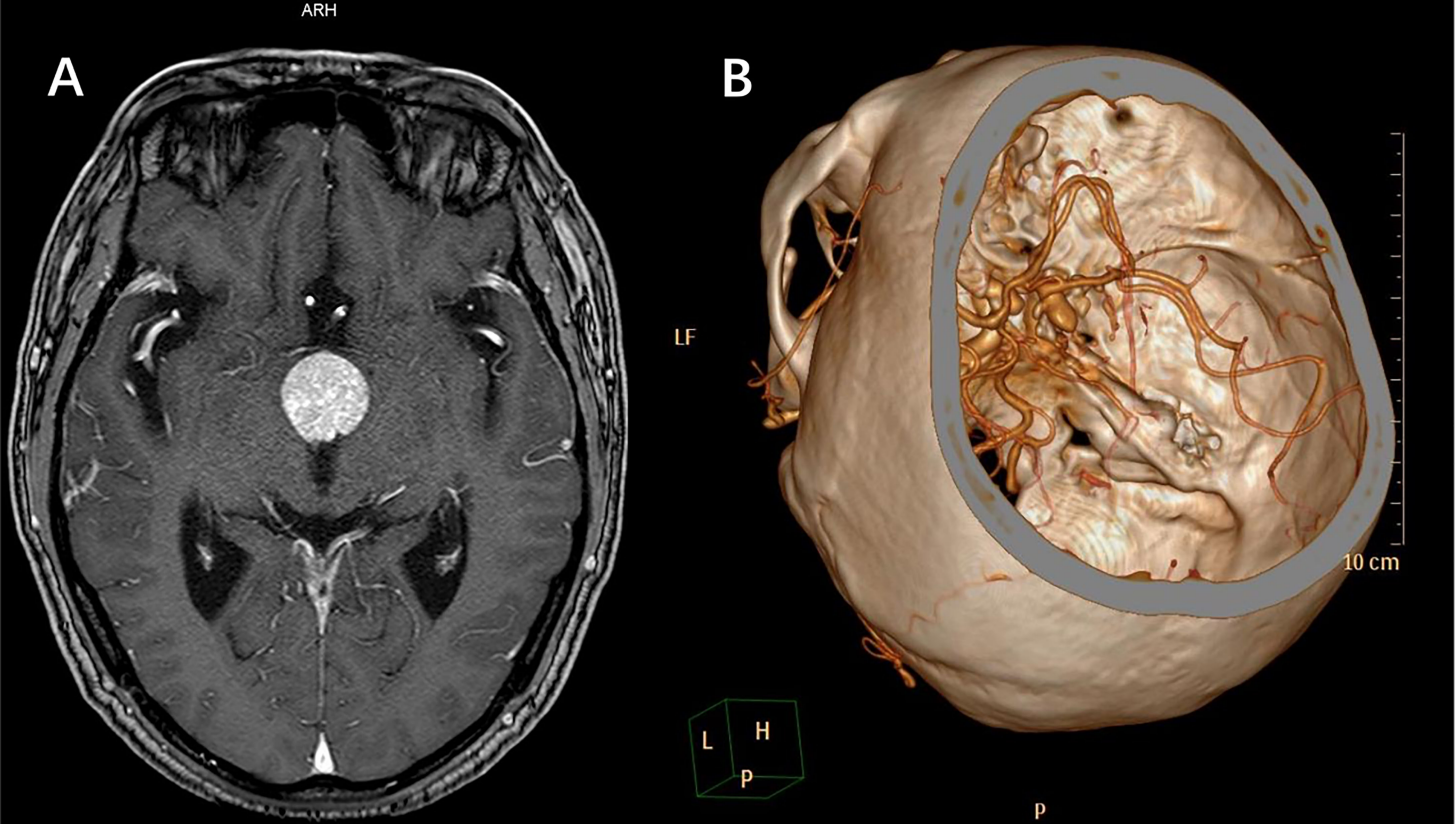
A case of typical radiological profile of sellar region lesion complicated with IAs. (A) MRI indicated sellar region lesion. (B) Further CTA revealed a partially thrombotic pseudoaneurysm formed from ruptured IA, and the IA located close to the sellar region lesion. In such occasion, incomplete preoperative examinations without CTA can lead to intraoperative massive hemorrhage, even perioperative death.
